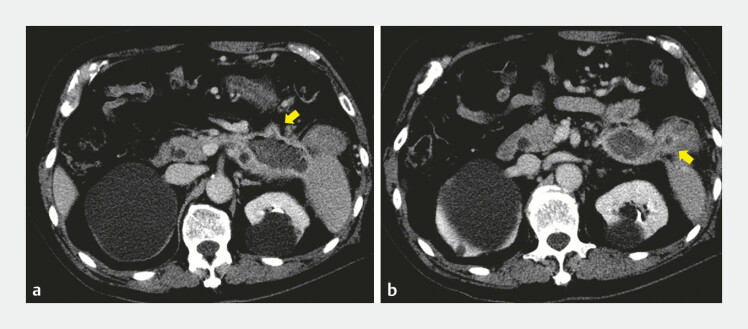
Computed tomographic images demonstrating fistulous communication between the walled-off necrosis and the small intestine (
